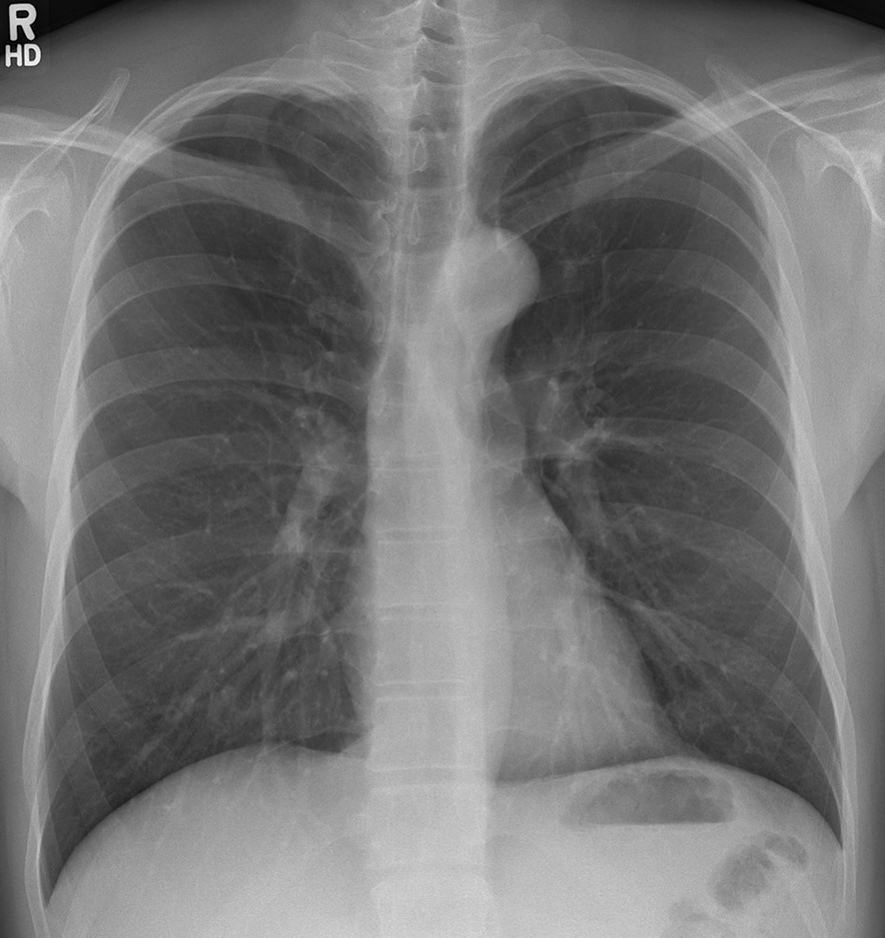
Chest radiograph (posteroanterior projection).
